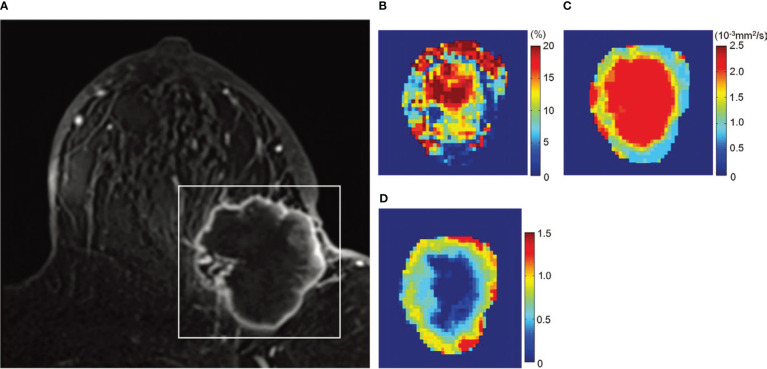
Example of non-Gaussian diffusion MRI maps in breast lesions. Images in a 72-year-old woman with invasive ductal carcinoma. (A) Dynamic contrast-enhanced axial MR image, (B) f_IVIM_ map, (C) ADC_0_ map, and (D) K map. The white rectangle on (A) shows the area covered by the parametric maps. (B) f_IVIM_ distribution is heterogeneous inside the tumor. The peripheral area of the tumor exhibits low ADC_0_
